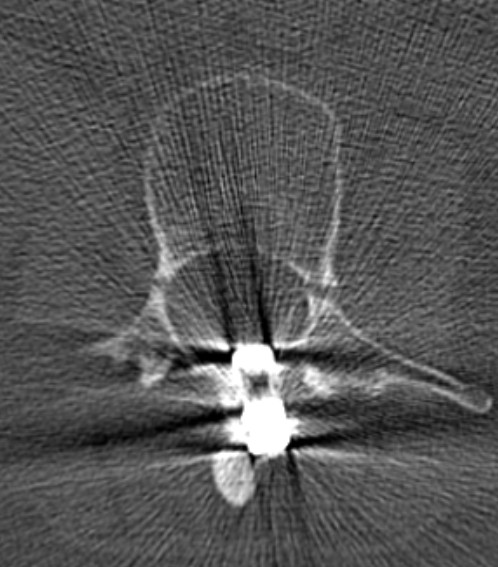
A computed tomography scan shows the fractured pedicles of L3
